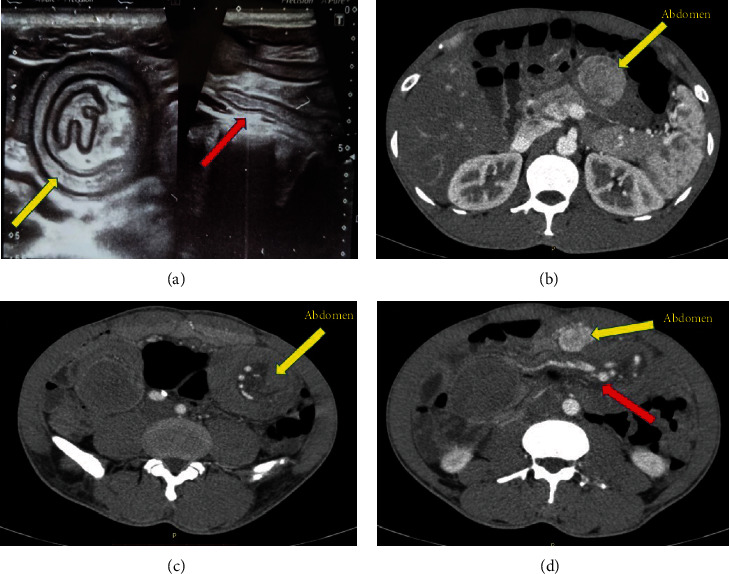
(a) Ultrasound scan showing a small bowel intussusception with the classical target sign (yellow arrow) and longitudinal section shown by red arrow. (b) Contrast enhanced computed tomography (CECT) of abdomen showing a mass (yellow arrow) attached to the proximal mesentery with arterial enhancement. (c) CECT showing proximal bowel intussusception (yellow arrow). (d) CECT showing proximal bowel intussusception (red arrow) and a mass attached to the proximal mesentery (yellow arrow).
